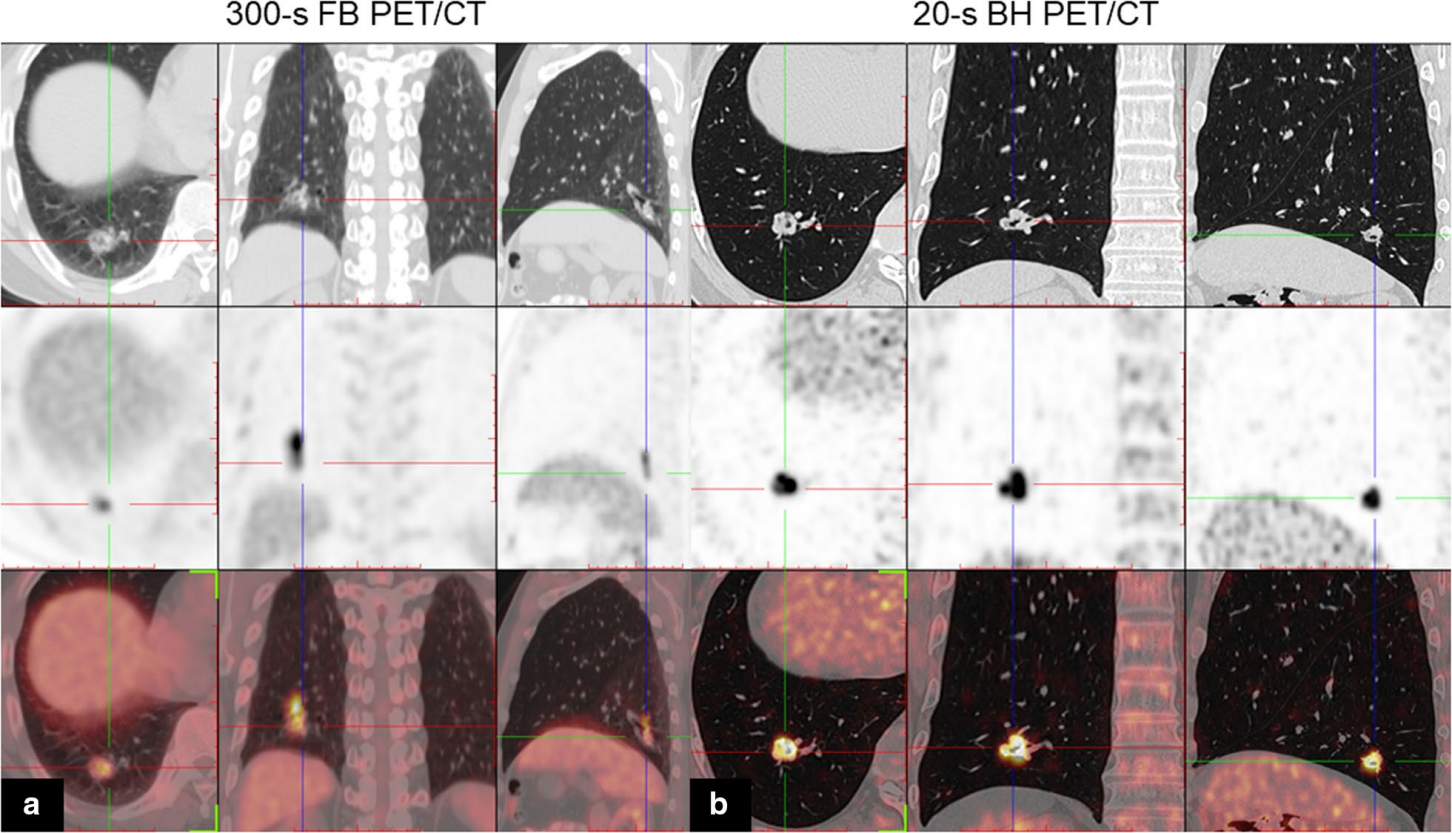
59-year-old man with lung adenocarcinoma. Axial, coronal, and sagittal chest CT (top row), PET (middle row) and PET/CT (bottom row) images of solitary solid lung nodule in the right lower lobe. a FB PET/CT; b BH PET/CT. Notice how the uptake area appears blurred owing to breathing artifacts in the FB PET image
